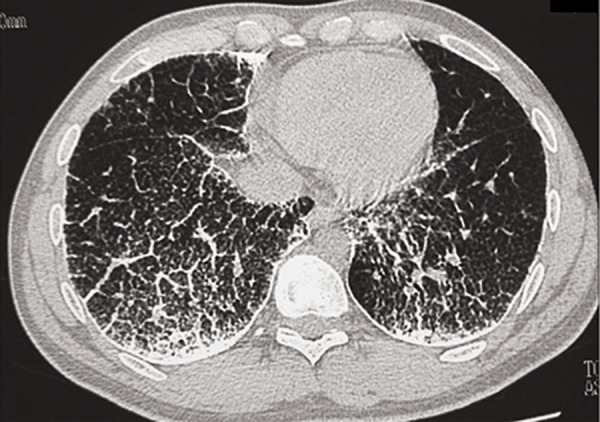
Chest HRCT: bilateral calcific micronodules diffusely involving the parenchyma and diffuse interstitial pattern ( septal thickening ). HRCT; High resolution computerized tomography scan.
